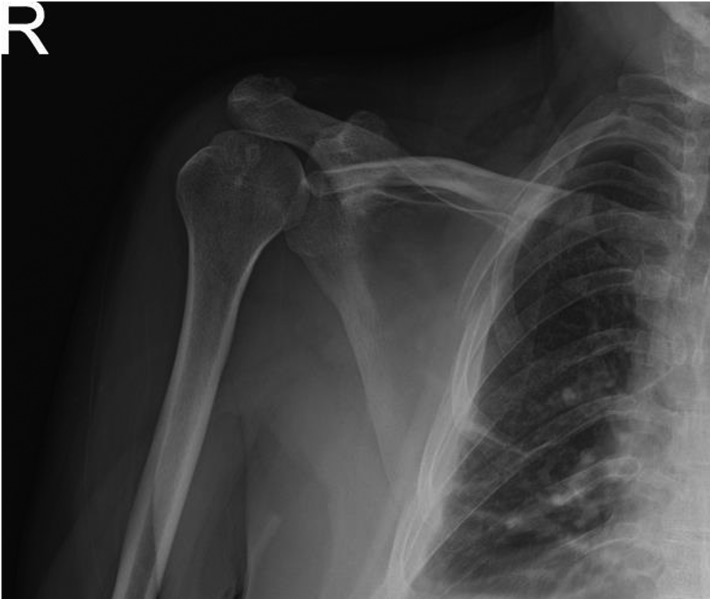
AP radiographs of the right shoulder 8 weeks after injury demonstrating subcoracoid dislocation of the distal clavicle.
